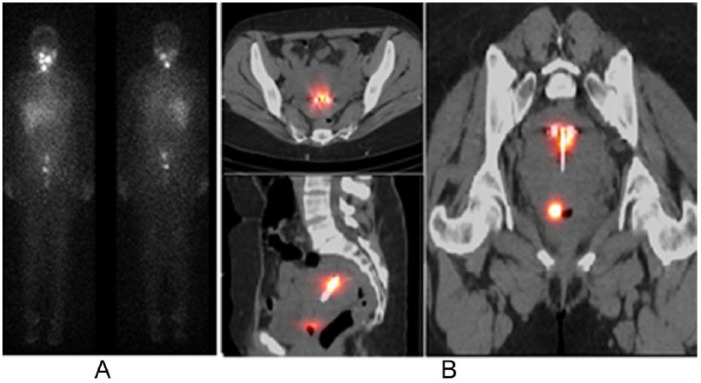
Inflammation around an intrauterine device and at a menstruating uterus/cervix as seen on WBS and SPECT/CT. A 41-year-old woman with a history of PTC was treated with surgery and then 150 mCi of I-131. Posttherapy WBS (5A) demonstrates 2 foci in the pelvis that on SPECT/CT (5B) were confirmed to represent accumulation of I-131 in the uterine cavity associated with an intrauterine device and at the cervix. Abbreviations: WBS, whole body scan; SPECT/CT, single-photon emission computed tomography.
